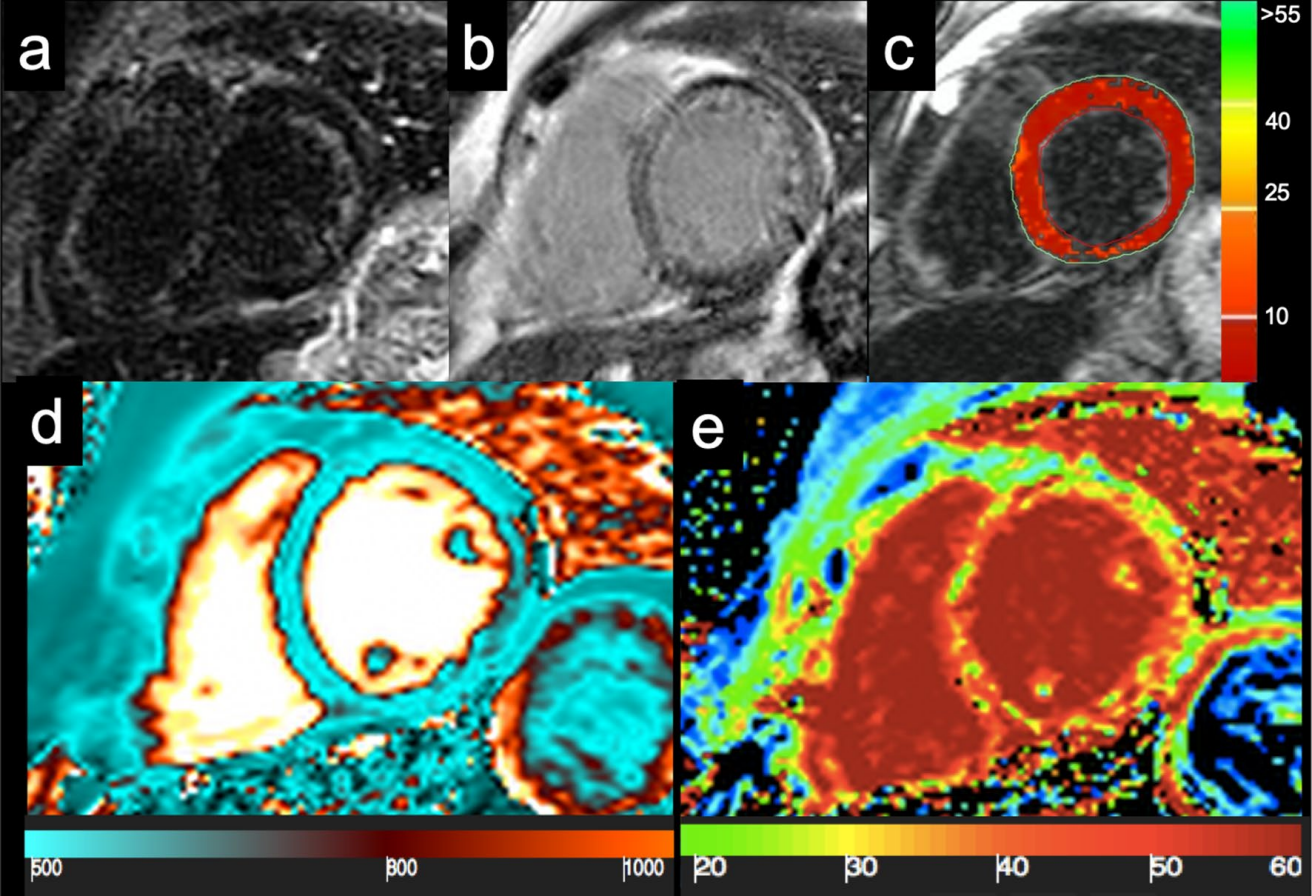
Cardiac iron overload—A 42-year-old woman with Cooley’s disease and moderate reduction in ventricular function (EF: 42%) show a global myocardial hypointensity on STIR image (a) and a diffuse inhomogeneous abnormal signal on LGE imaging (b), with no evidence of clear focal areas of enhancement. Analysis of T2* map (c), generated by traditional multiecho gradient echo T2-weighted sequence, shows a diffuse and marked reduction in the global myocardial T2* relaxation time (T2* = 0–1.5 ms, normal value > 20 ms). nT1 map (d) shows a significant reduction in global nT1 value (nT1 ≈ 535 ms, normal value 970–1020 ms), affected by susceptibility effect of intramyocardial iron accumulation. ECV map e reveals diffuse fibrosis (ECV ≈ 38–40%). *STIR* short tau inversion recovery; *LGE* late gadolinium enhancement; *nT1* native T1 value; *ECV* extracellular volume fraction
